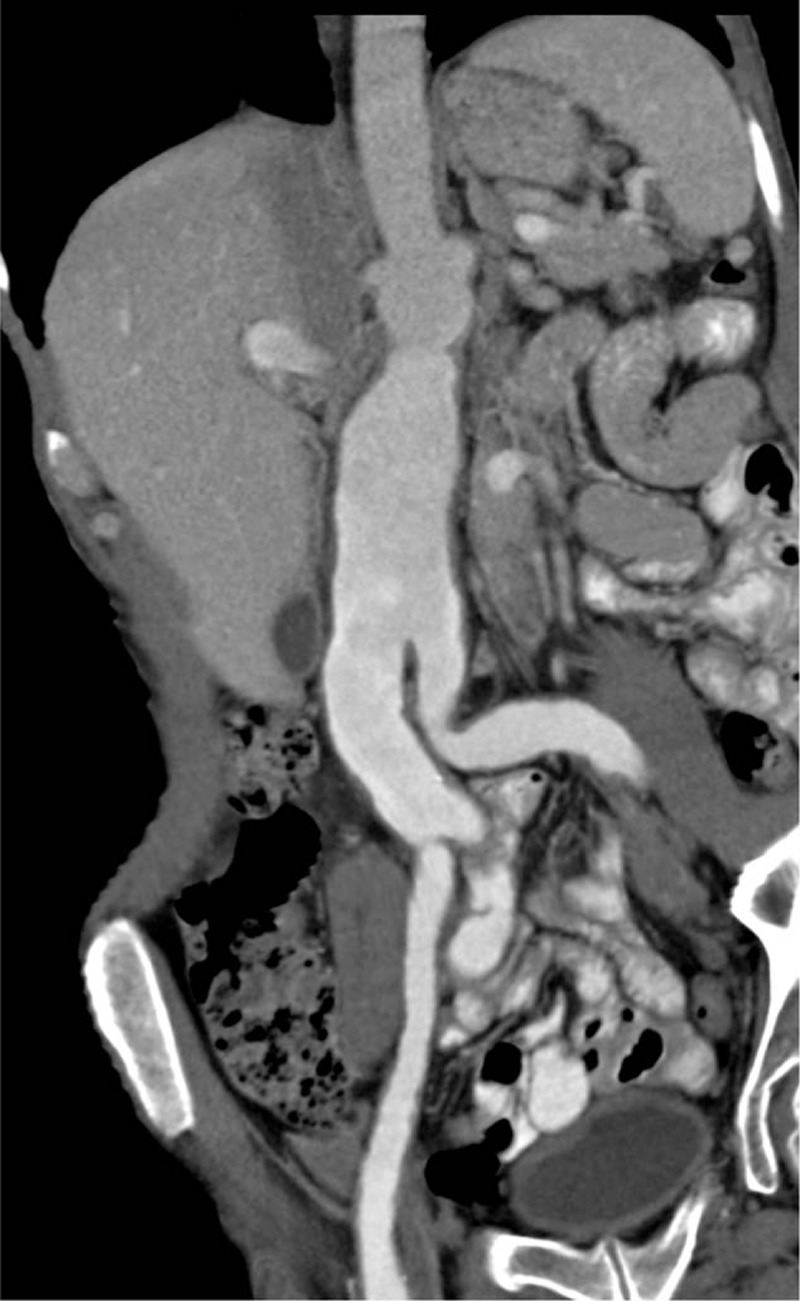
CT scan of the abdomen showing diffuse, nonatherosclerotic arterial disease with aneurysmal dilatation of the abdominal aorta and common iliac arteries.
